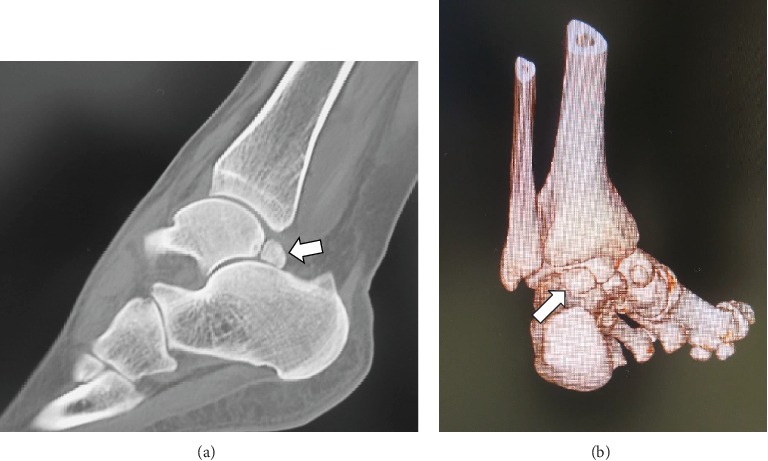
Computed tomography images showing the os trigonum connected to the posterior aspect of the talus. (a) Plain image, sagittal view (arrow). (b) Three-dimensional image, posterior view (arrow).
